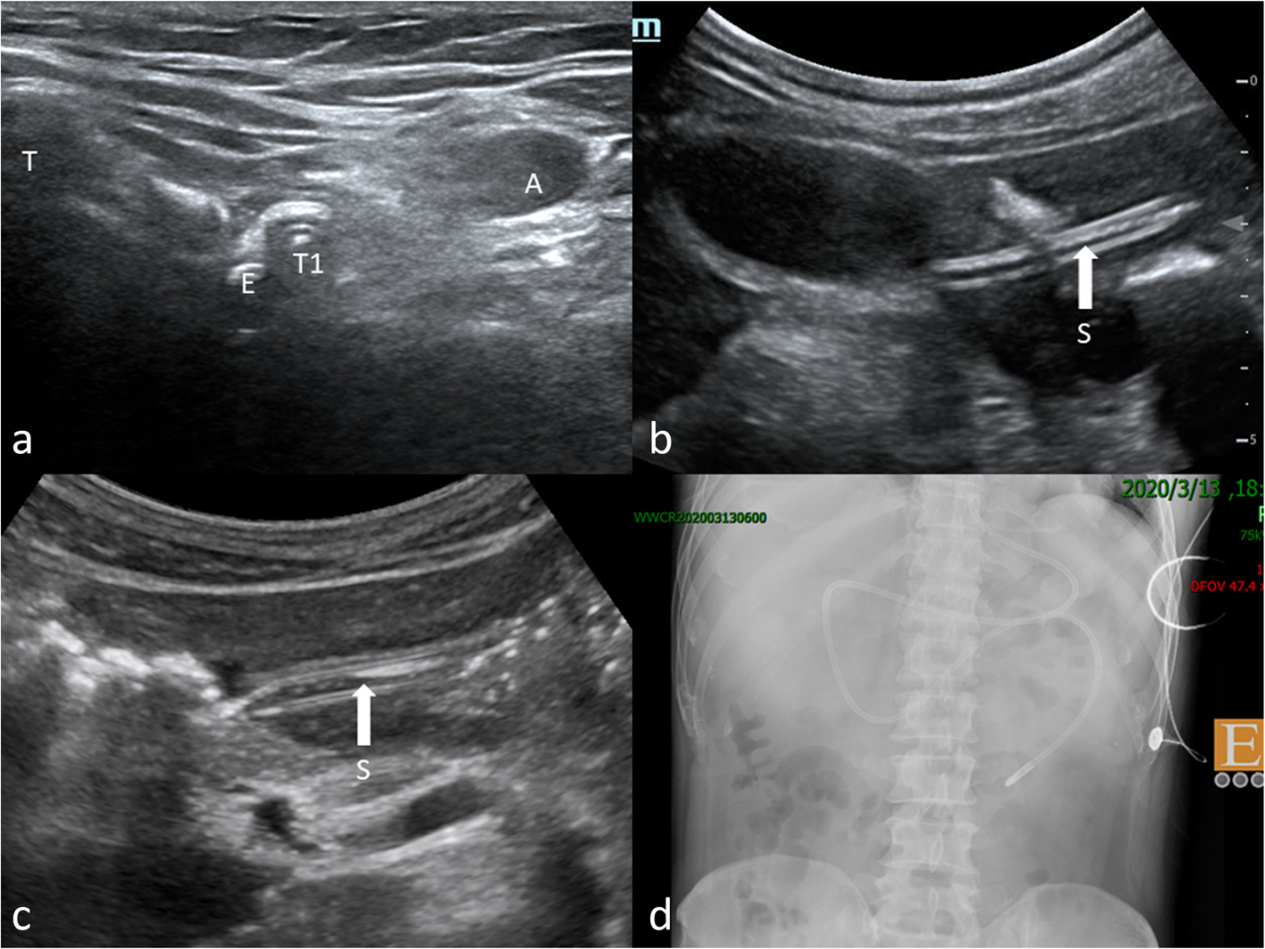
The location of the trachea (T), carotid artery (A), nasointestinal tube (T1), and esophagus (E) (a). Tram-track signs with stylet (S) in the longitudinal section (b). The stylet (S) tip in pylorus in the longitudinal section (c). Abdominal plain radiograph showed the tip of the tube (d)
